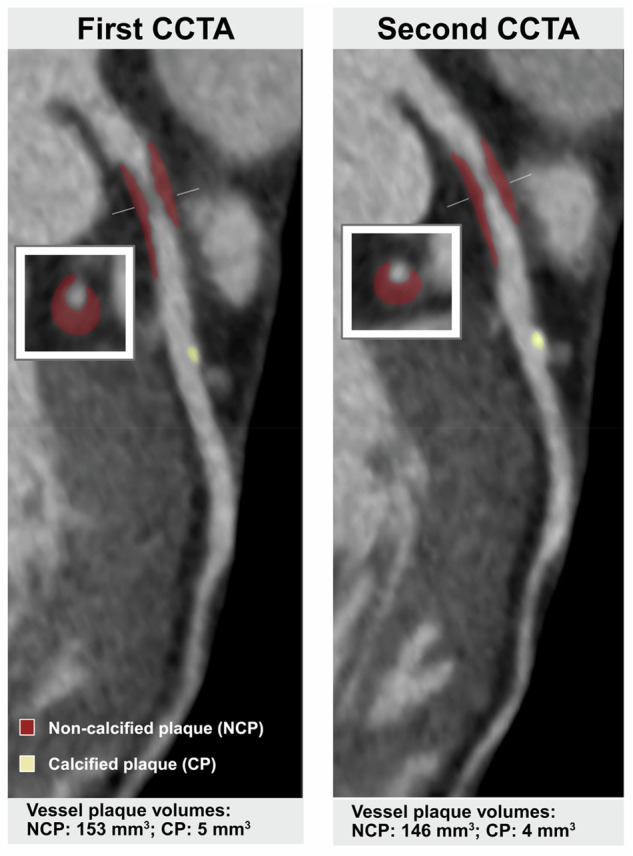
Example of plaque adjudication side-by-side in one vessel. CCTA, cardiac CT angiography
